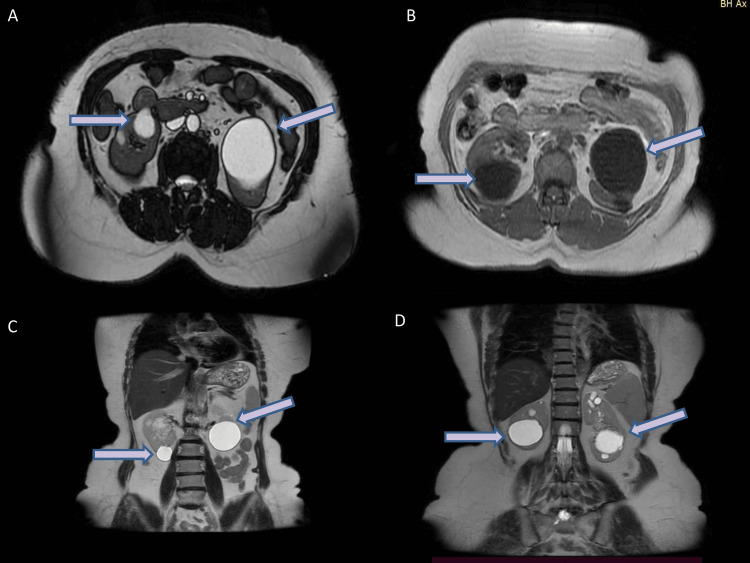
Magnetic resonance imaging of the abdomen Axial (A, B) and coronal (C, D) T2-weighted MRI images show normal-sized kidneys and several round, thin-walled, homogeneous, fluid-filled cysts (arrows), distributed throughout the renal parenchyma and located predominantly in the renal cortex.
